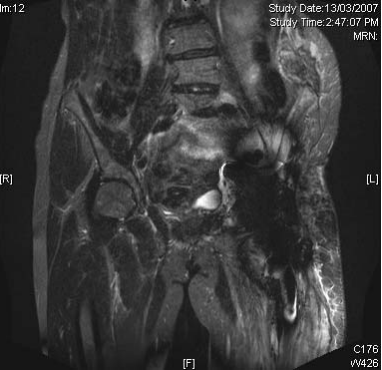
Pre-Operative MRI coronal view of Case Patient showing local cancer recurrence involving left pelvis and surrounding the saddle prosthesis.
